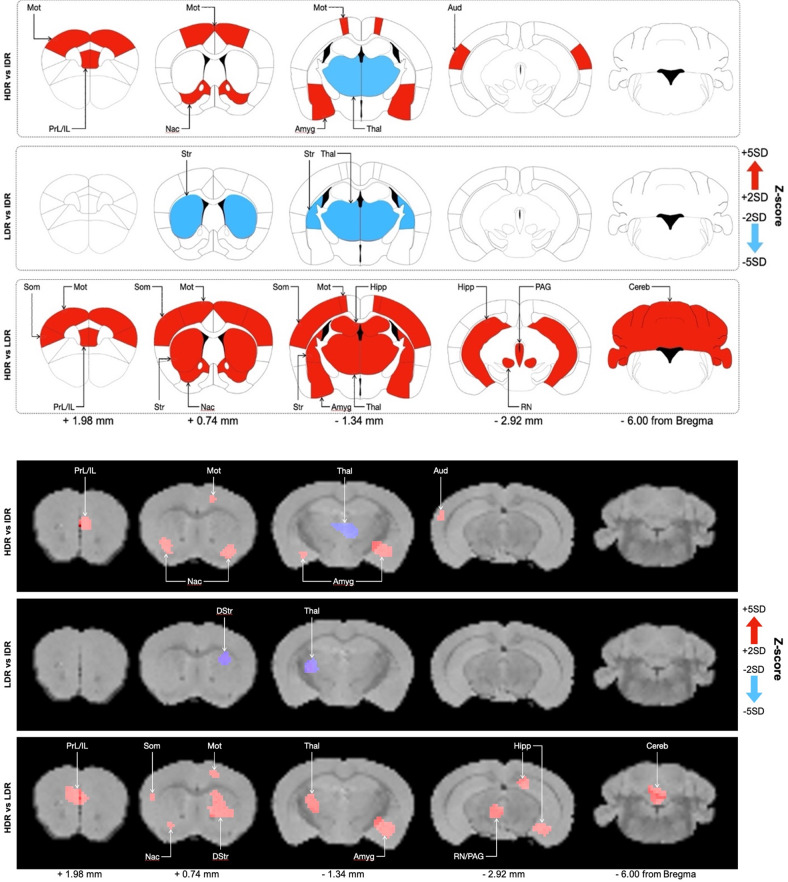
Comparisons of the changes in metabolic activity in the different phenotypes at PND 37. A Summary of the significant increases (red) and decreases (blue) in uptake observed in HDR vs LDR; HDR vs IDR and IDR vs LDR (*n* = 33) at PND 37 presented on representative coronal plates of the Paxinos and Watson atlas with *P* < 0.01. B Example of the significant differences in ^18^F-FDG uptake observed in HDR vs LDR; HDR vs IDR and IDR vs LDR (*n* = 33) at PND 37 presented on coronal images of Z-scores maps fused with an MRI template (increases in 18-FDG uptake in red, decreases in ^18^F-FDG uptake in blue). Aud dorsal secondary auditory cortex, Thal thalamus, Amyg amygdala, Hipp hippocampus, PAG periaqueductal gray, RN red nucleus, DStr dorsal striatum, Str striatum, IL infralimbic cortex, PrL prelimbic cortex, Mot motor cortex, Som somatosensory cortex, Nac nucleus accumbens, cereb cerebellum, PND post natal day, HDR high depression risk, IDR intermediate depression risk, LDR low depression risk.
